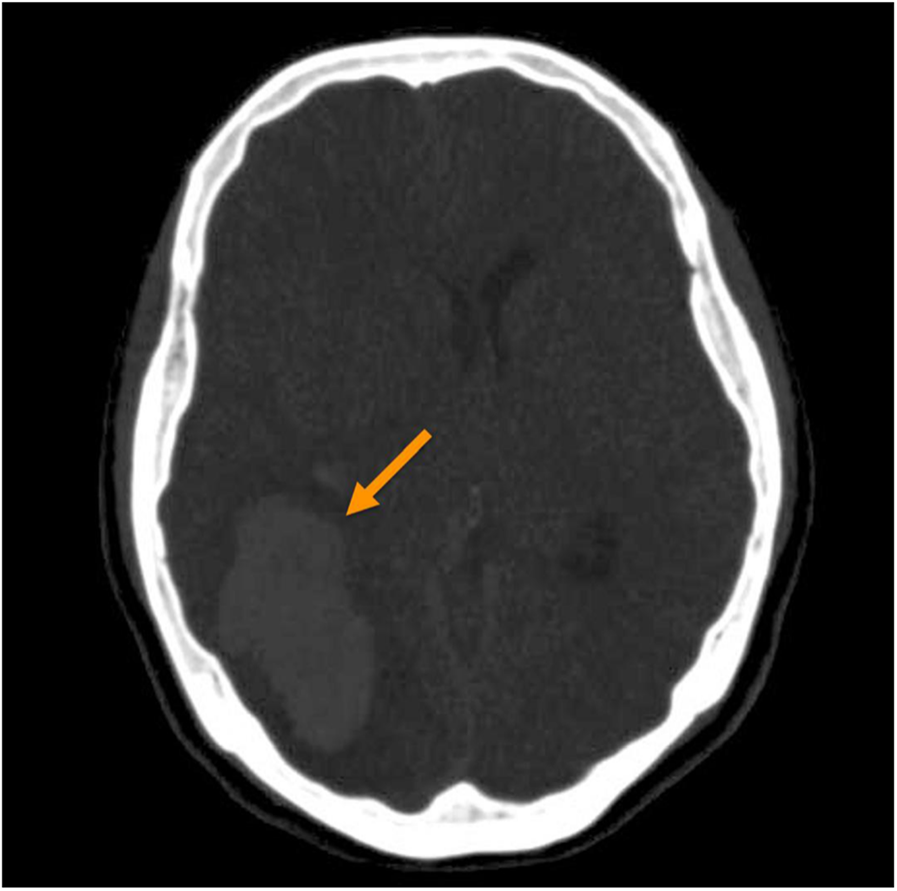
Non-enhanced brain computed tomography (CT). Brain CT showed an acute intracerebral hemorrhage (arrow) with perilesional edema at the parietotemporooccipital lobe. An acute intraventricular hemorrhage causing a midline shift to the left was also noted
